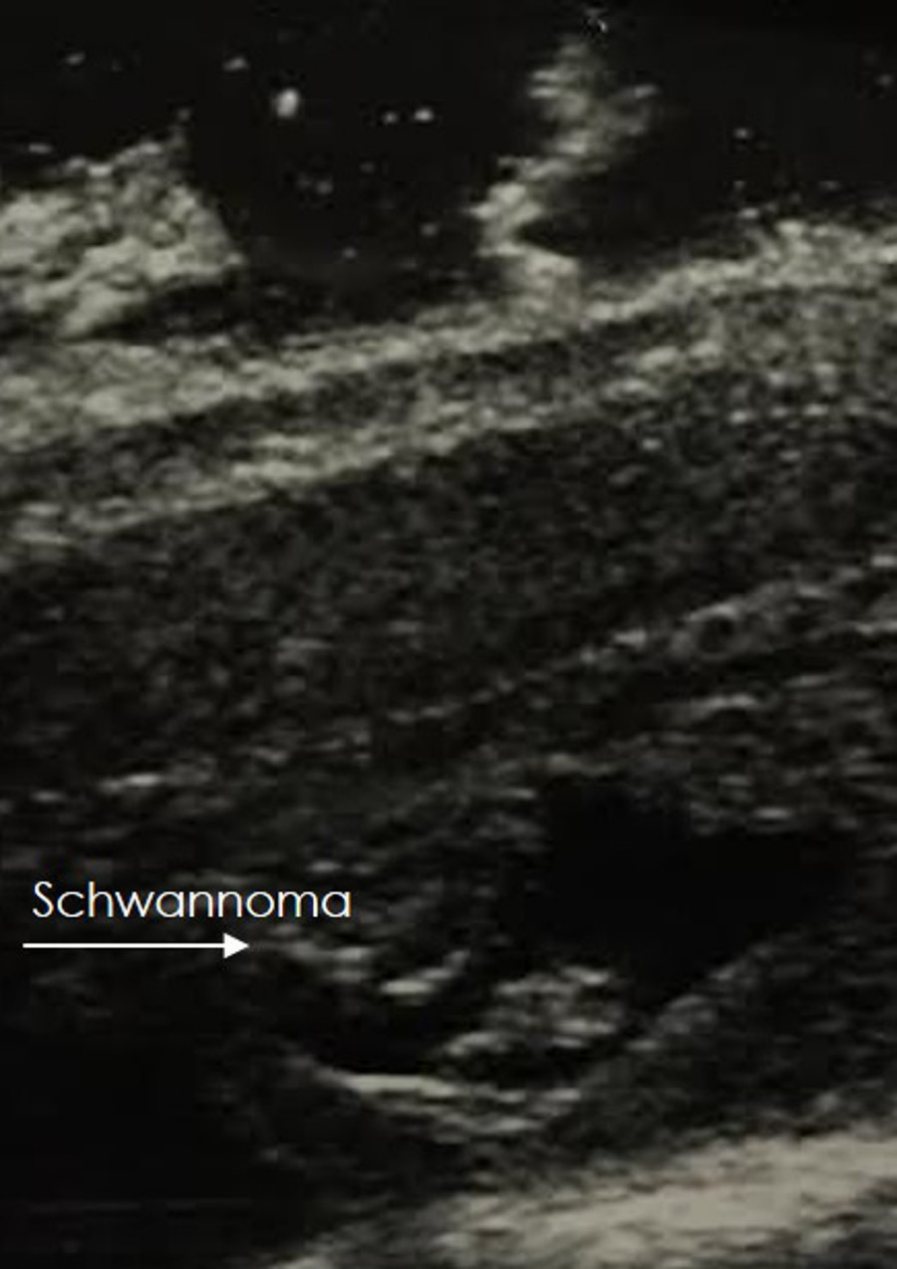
Intraoperative ultrasound An intraoperative ultrasound confirmed the location of the intradural lesion just prior to the opening of the dura.
